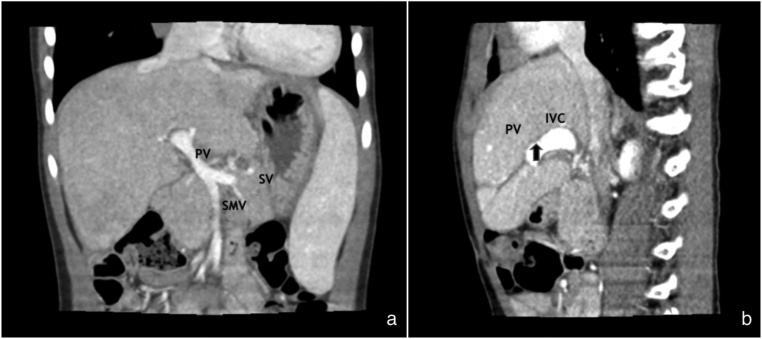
A contrast-enhanced CT scan at 6 months postoperatively showed disconnection between the left portal vein (PV) and IVC.
